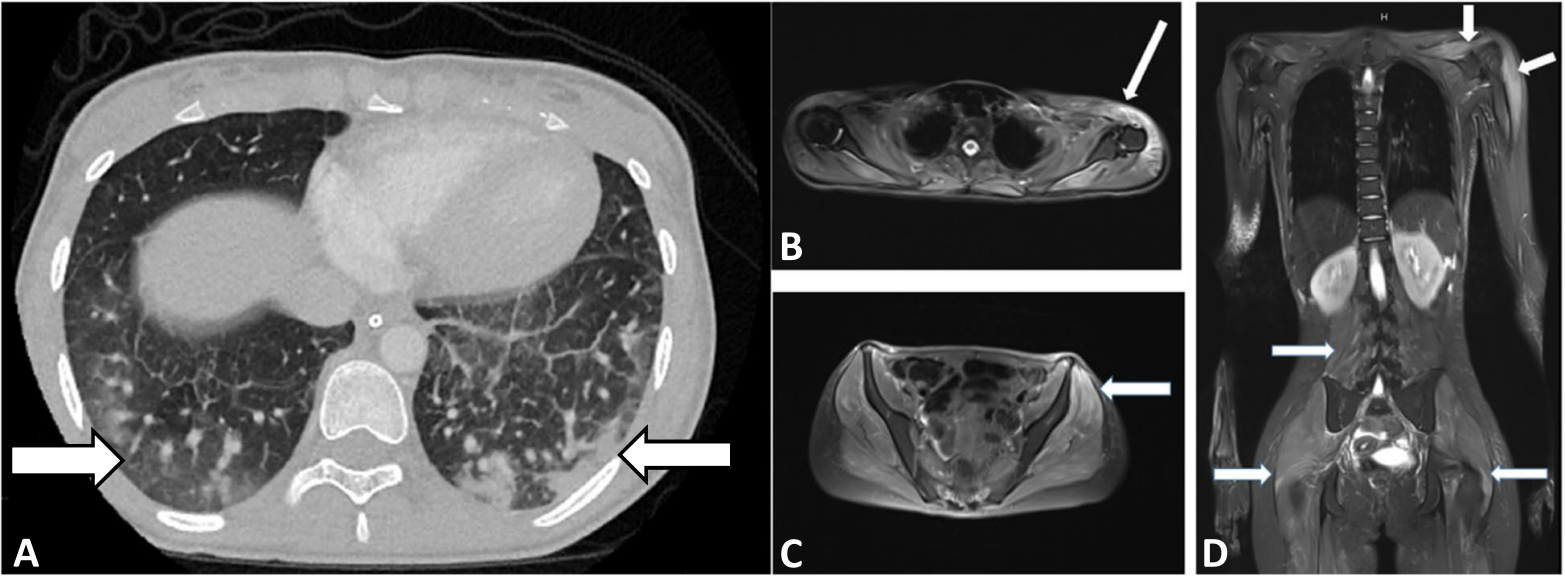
A CT chest showing bilateral posterior lower lobe consolidations worse on the left B. T2 fat saturated MRI showing myositis of the left deltoid C. Myositis of the gluteus medius and minimus D. Myositis of the shoulders
